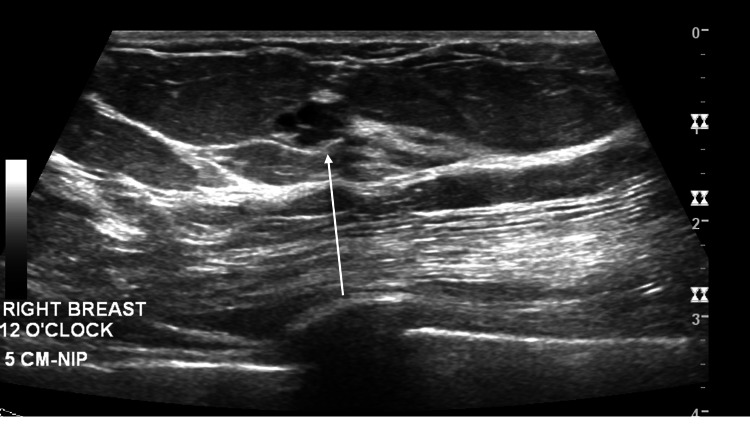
Ultrasonography of the right breast demonstrates a 7 x 7 x 5 mm solid and cystic hypoechoic mass with indistinct margins with no significant posterior features at 12 o’clock, 5 cm from the nipple
